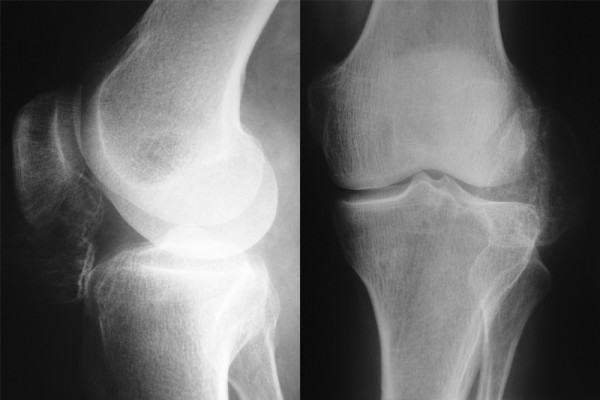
A and B. The osteochondral mass adjacent to patella in the left knee is seen at AP and lateral radiographs.
